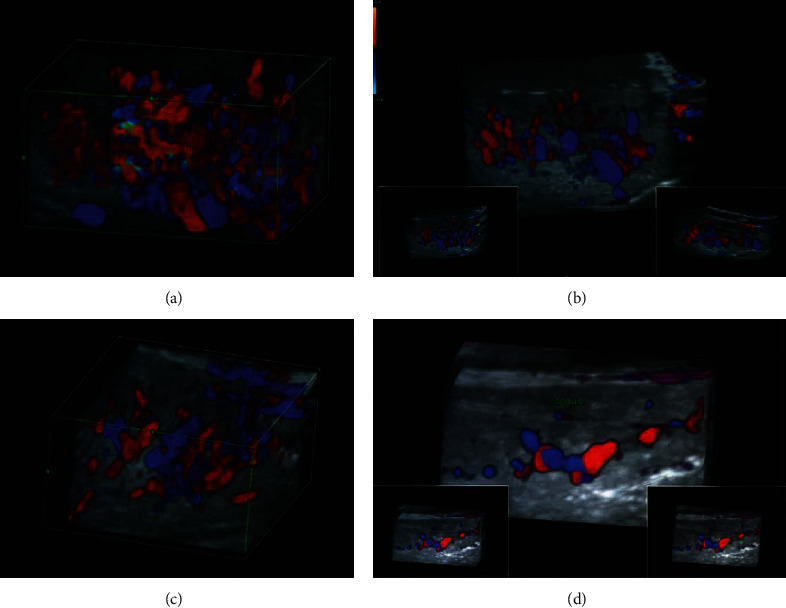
Color/power Doppler imaging of the AVM: (a) baseline; (b) 12-month follow-up; (c) 24-month follow-up; (d) 40-month follow-up.
